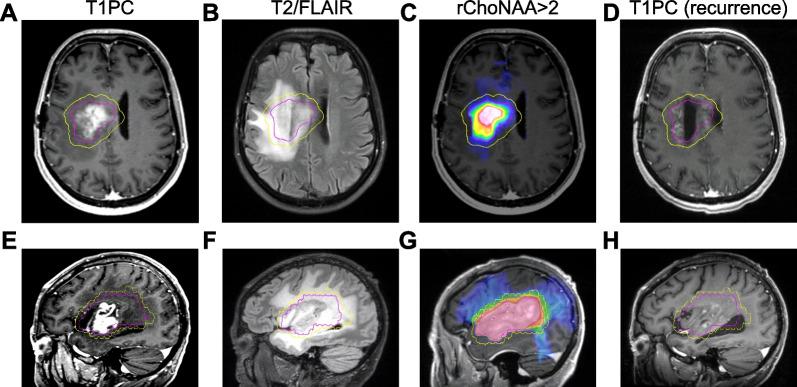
Two patients with recurrence within rChoNAA > 2 but outside of T1 post-contrast delineated GTV. A Axial pre-treatment T1PC from patient with rGBM treated with re-RT. Yellow outlines the CTV and magenta contour outlines the pre-treatment rChoNAA > 2 volume. B Pre-treatment T2/FLAIR. C rChoNAA > 2 overlaid pre-treatment T1PC. D Post-treatment T1PC. An area of recurrence is seen medially that was identified by the initial rChoNAA > 2 maps. E Sagittal pre-treatment T1PC from a separate patient with rGBM treated with re-RT. Yellow outlines the CTV and magenta contour outlines the pre-treatment rChoNAA > 2 volume. F Pre-treatment T2/FLAIR. G rChoNAA > 2 overlaid pre-treatment T1PC. H Post-treatment T1PC. Area of recurrence posteriorly was largely included in the rChoNAA > 2 volume but not T1PC volume. Note that areas of recurrence are not avidly enhancing due to concurrent bevacizumab administration
